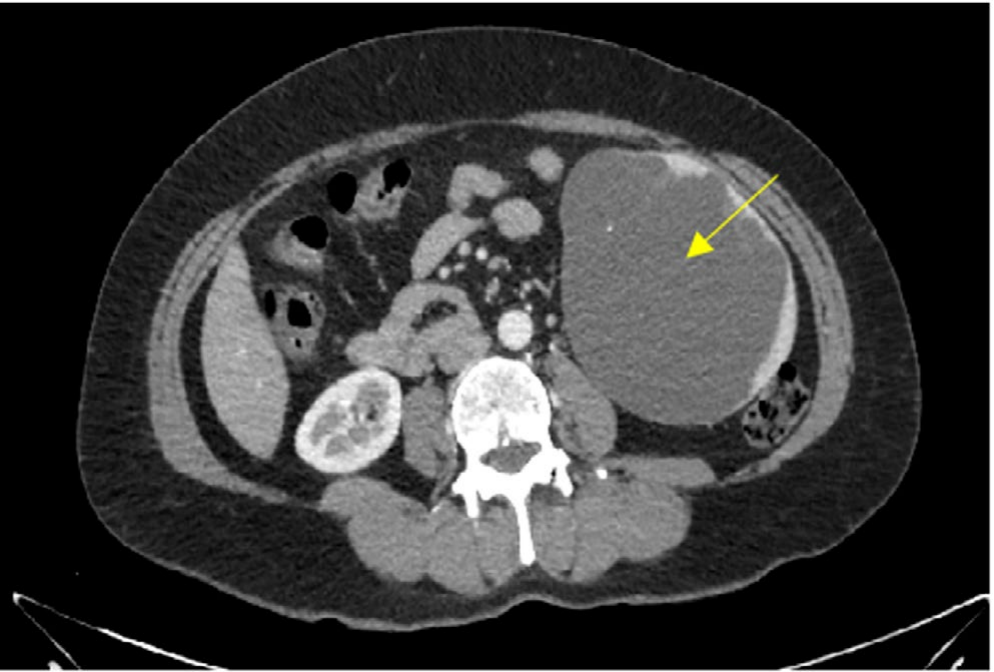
Axial abdominal CT revealed a large cyst (yellow arrow) located at the lower pole of the spleen.
